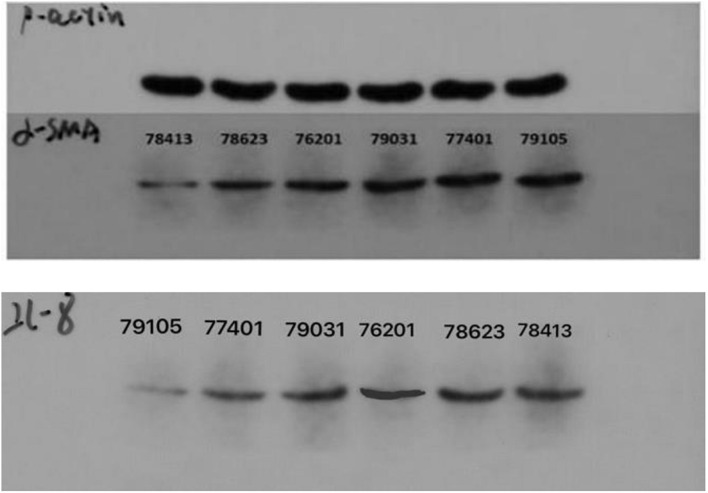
The electrophoretogram of *ACTA2 (α-SMA)* and *CXCL8 (IL-8)* at CT-LCG and FT-LCG.
